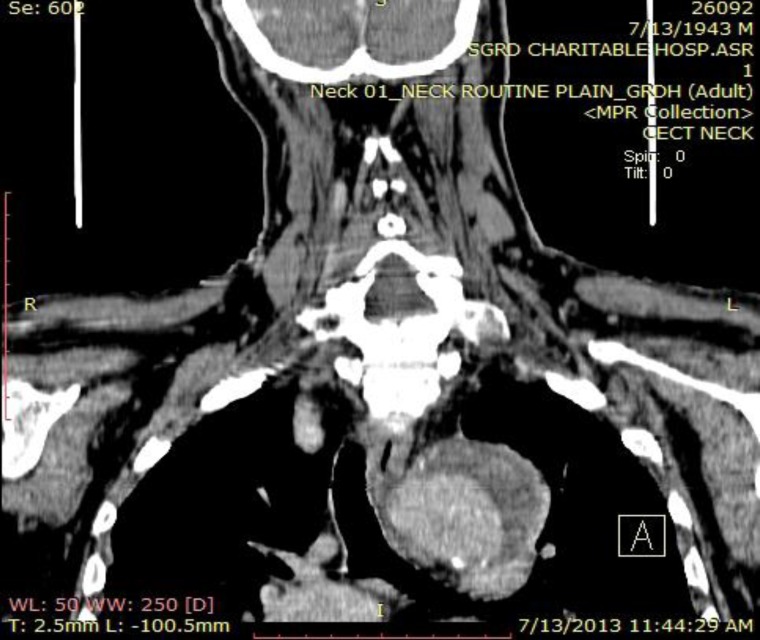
Contrast enhanced computed tomography scan shows crescent shaped hypo attenuating area of size 6.8x1.8 cm along the left wall of aortic arch between the left common carotid and extending up to the left subclavian vessel with atherosclerotic plaque
